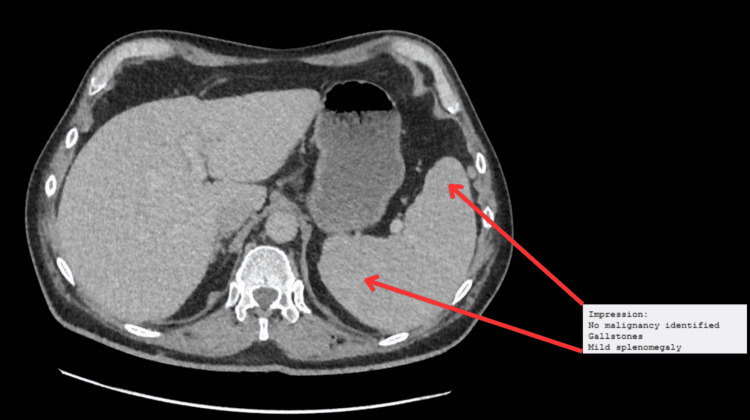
Mild splenomegaly in CT-CAP CT-CAP: computed tomography of the chest, abdomen, and pelvis
